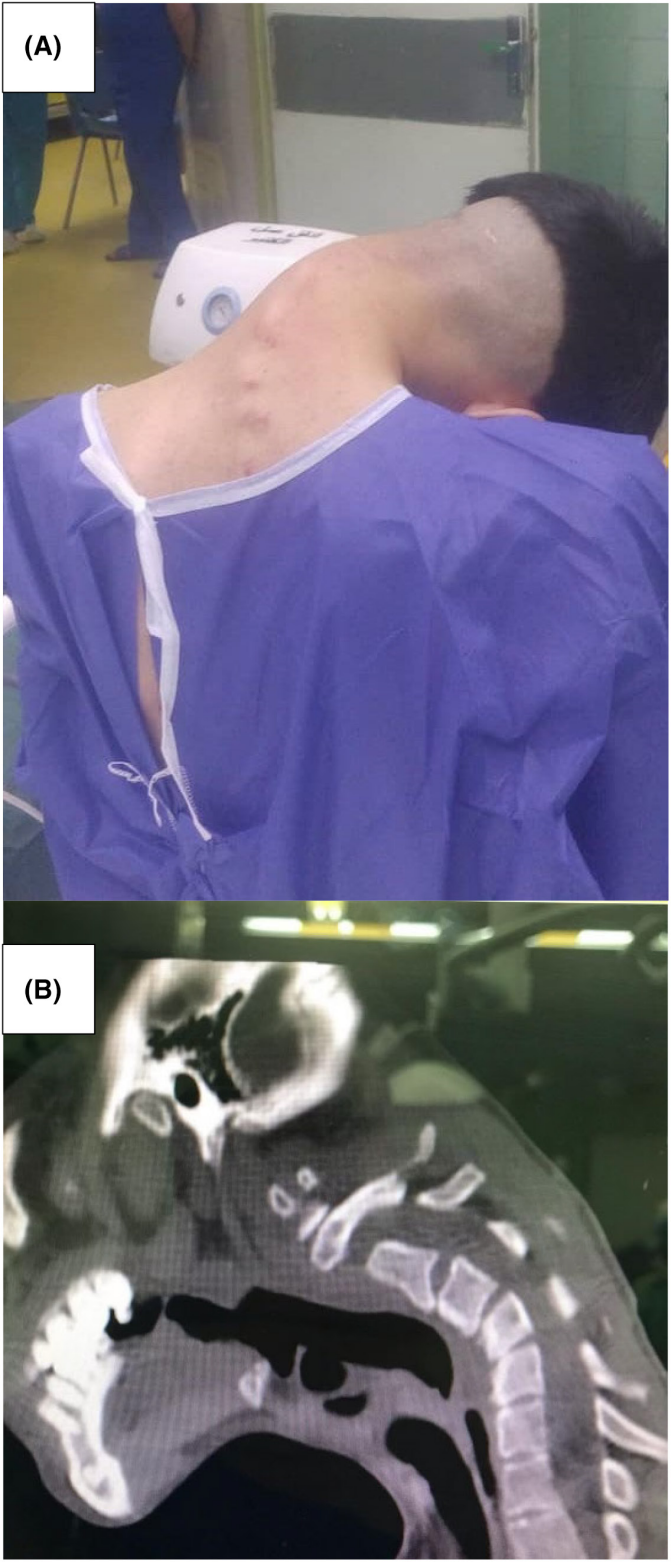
(A) Progressive cervical spine deformity in a 23‐year‐old previously healthy male due to substance abuse. (B) The CT scan of the patient reveals severe neck kyphoscoliosis.
